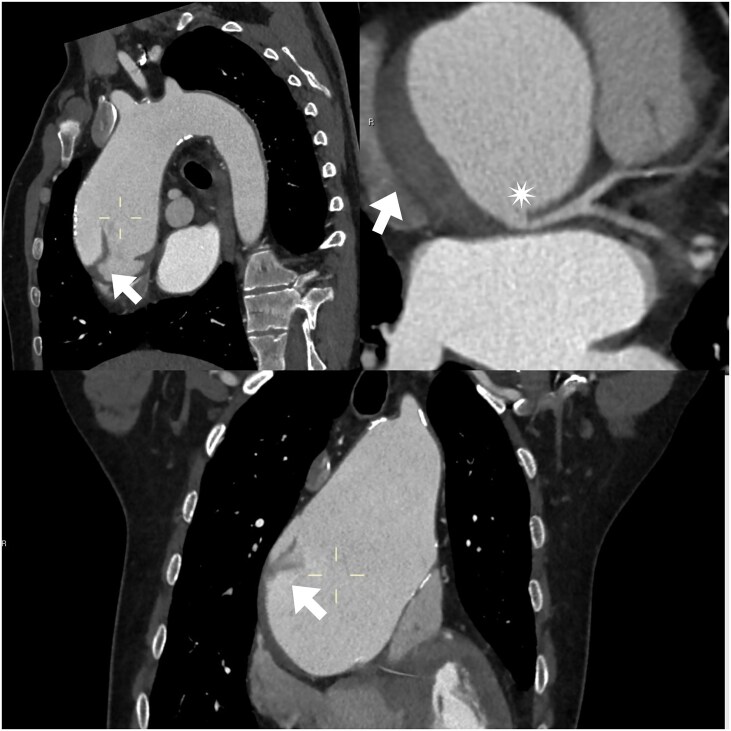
CT angiography image (sagittal, oblique and coronal). Giant ascending aortic aneurysm with Stanford type A dissection. It shows (arrows) the beginning and end of the dissection flap, without involvement of the LCA origin (star) or supra-aortic trunks.
